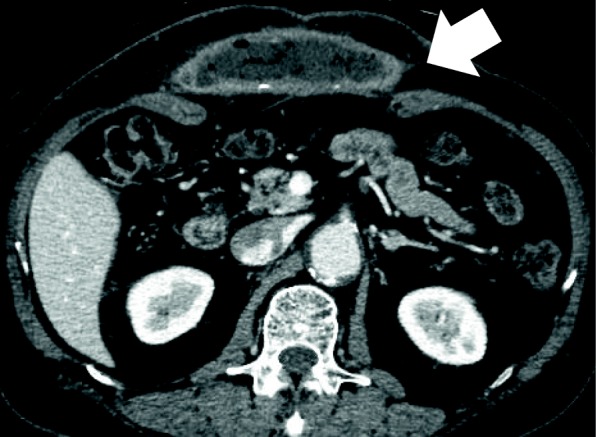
Preoperative CT suggesting subcutaneous encapsulated 12 × 6.0 × 2.5 cm abscess formation (arrow)
